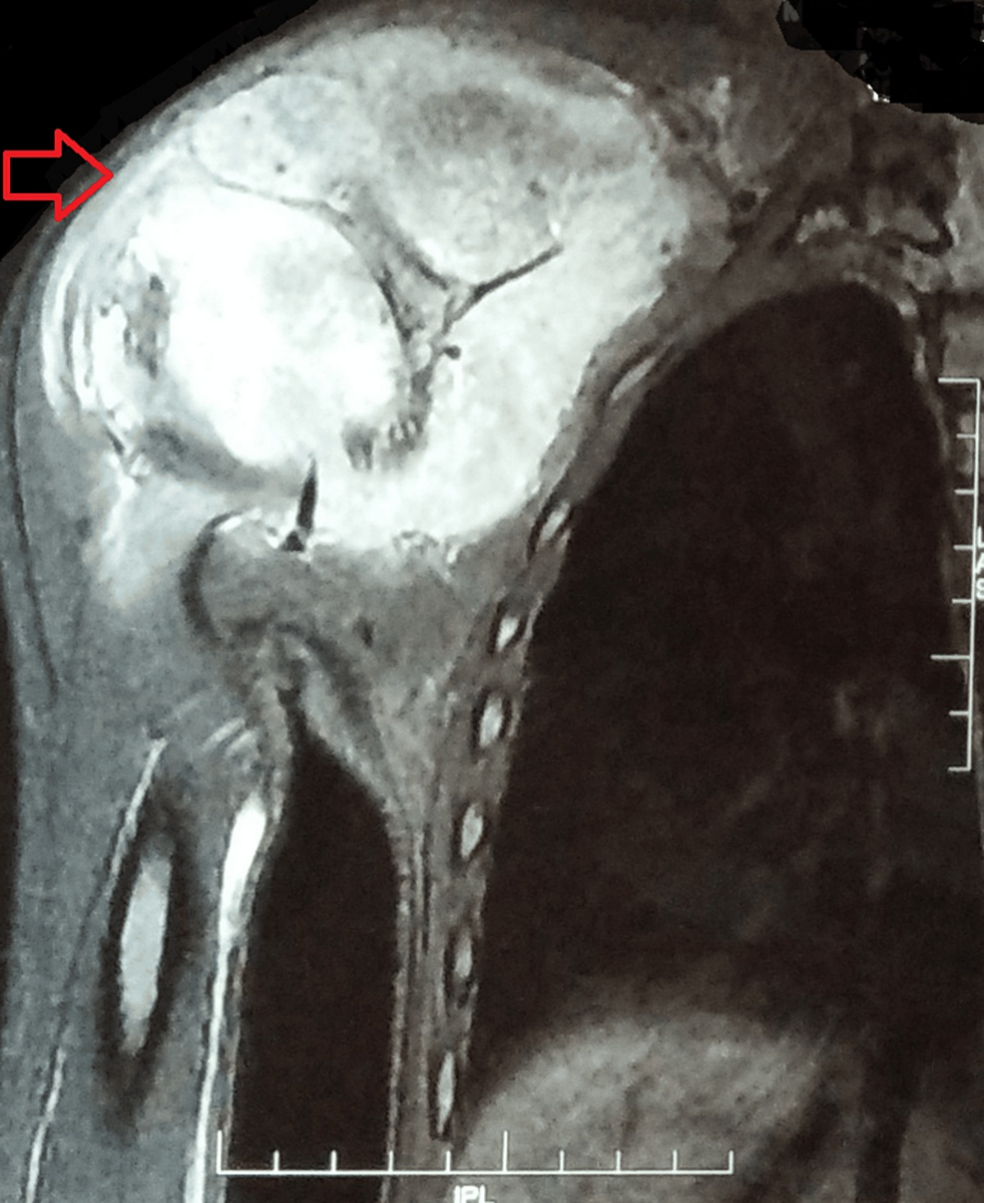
An MRI with contrast suggestive of a lobulated mass lesion on the ventral and dorsal aspects of the right scapula, with evidence of destruction of the body of the scapula. It also shows a focal bony lesion in the right proximal humeral meta-diaphysis MRI: magnetic resonance imaging
